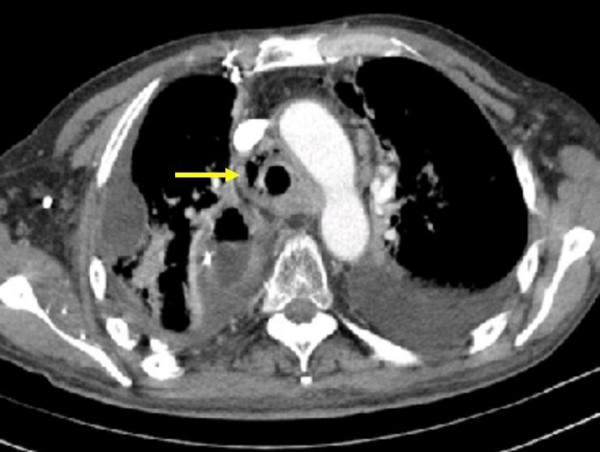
Axial contrast enhanced CT image showed a mediastinal tumor with mediastinal air and a perforation of the tracheal wall (arrow).
